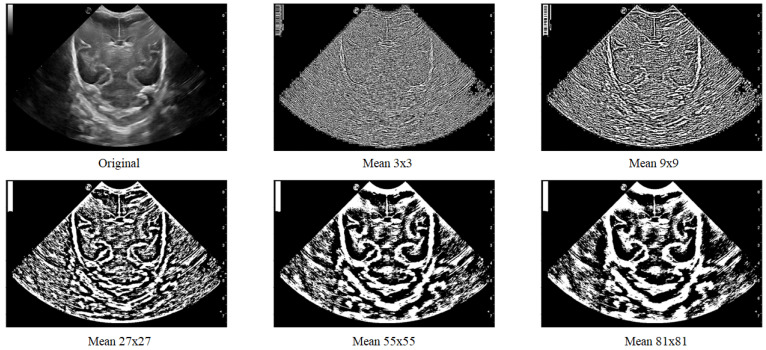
Local filter has been applied to an ultrasound image (Original) with different surface sizes for analysis. As shown in the figure, the resulting images obtained using Mean 3 × 3, 9 × 9, 27 × 27, 55 × 55, and 81 × 81 pixels are presented from left to right as an example.
